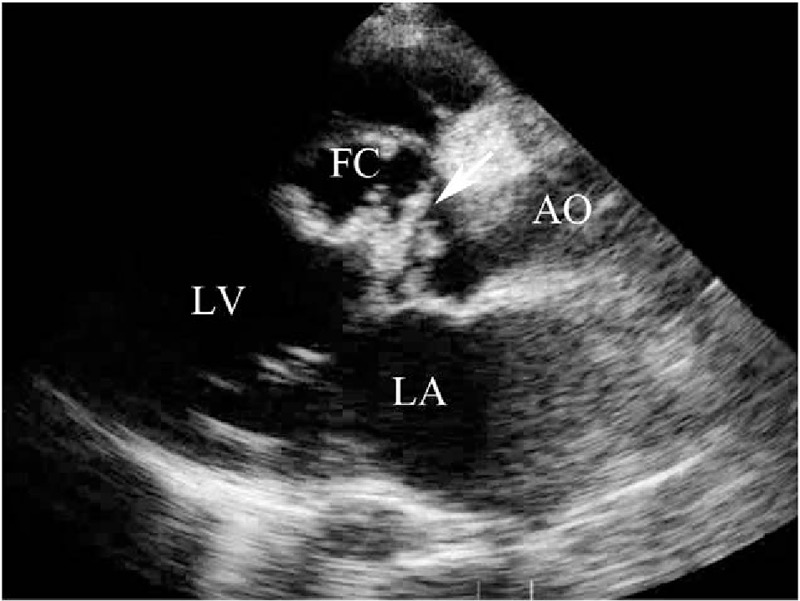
(Patient #7) Transthoracic echocardiogram (parasternal long axis view) showing thickened aortic valve leaflets with vegetations and the entry site of rupture (arrow) from the right sinus of Valsalva into the dissecting cystic-like false cavity in the interventricular septum (see also video 1). AO = aorta, FC = false cavity, LA = left atrium, LV = left ventricle.
